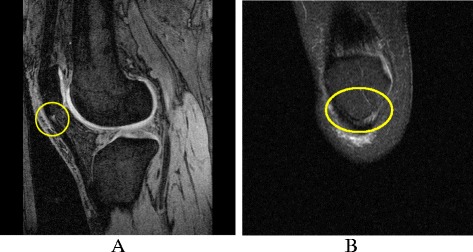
Patellar tendinopathy on MRI. a Sagittal T1-weighted fat-saturated image showing patellar tendinopathy. b Coronal T2-weighted fat-saturated image showing patellar tendinopathy
